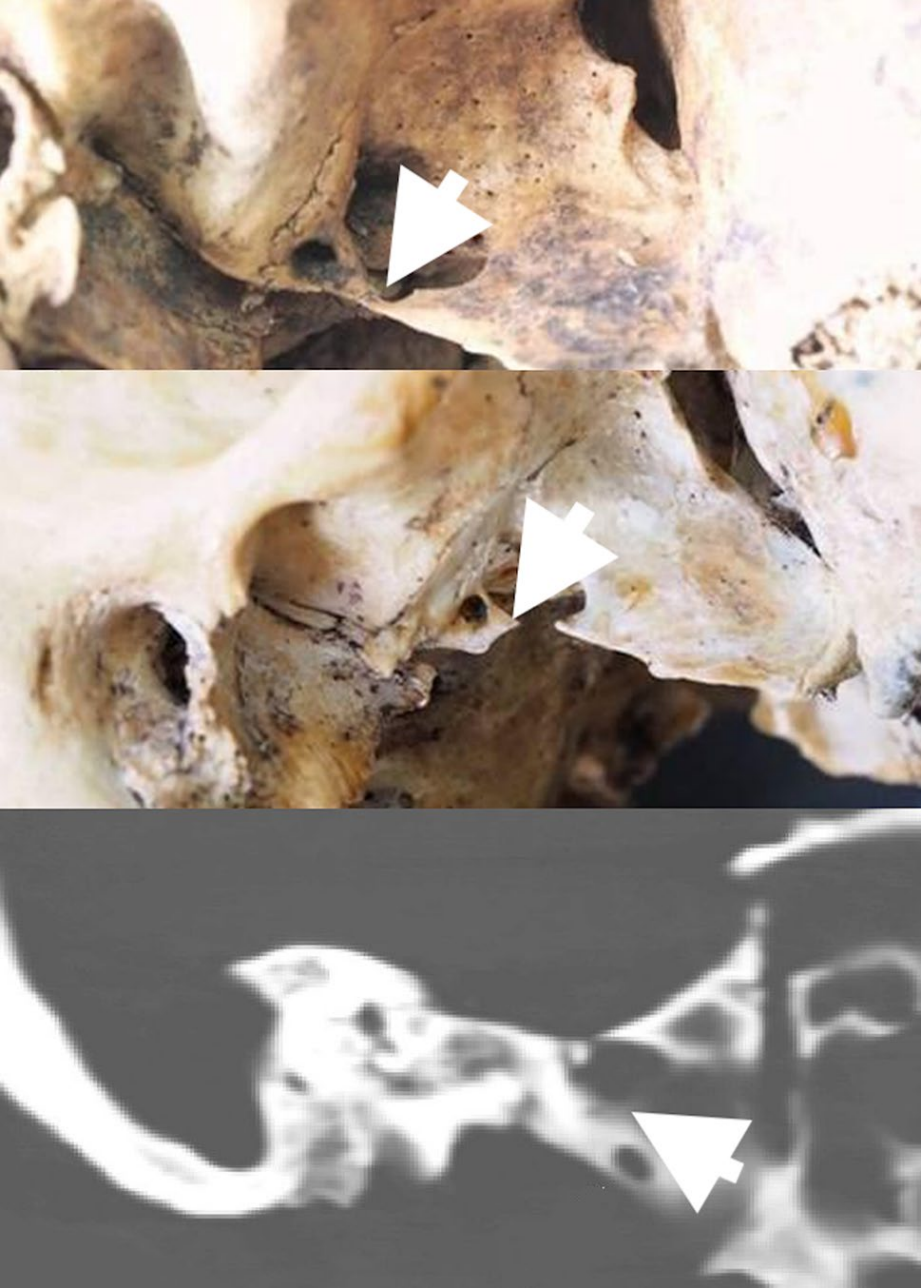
Lateral view of the dry skulls (a, b), and CT scan (c) with presentation of the a, c complete, and b incomplete pterygospinous bar (both marked with white arrows); *ANT* anterior, *POST* posterior
